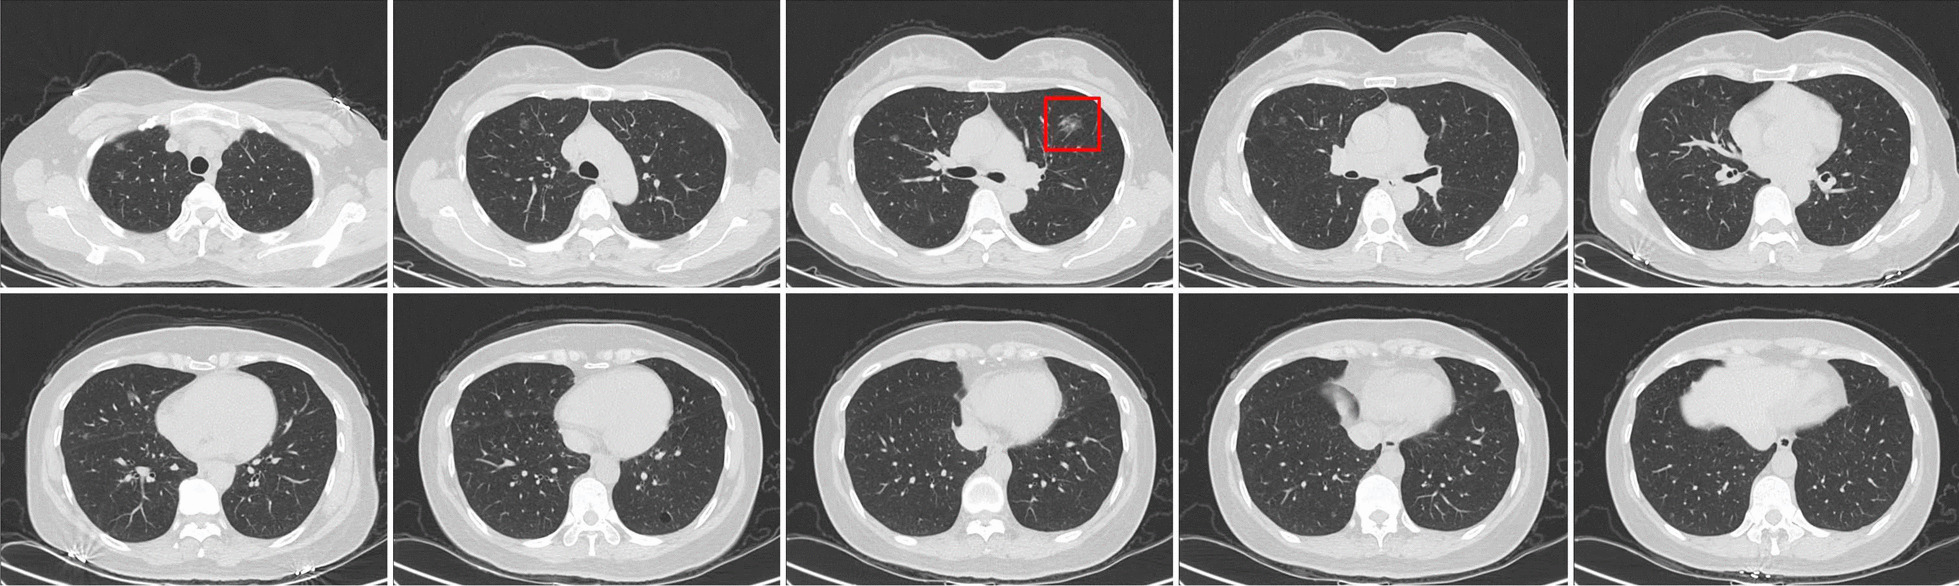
The preoperative computed tomography scanning. A 1.5-cm diameter irregularly shaped lesion in the left upper lung, and mGGNs in all lobes of the right lung
